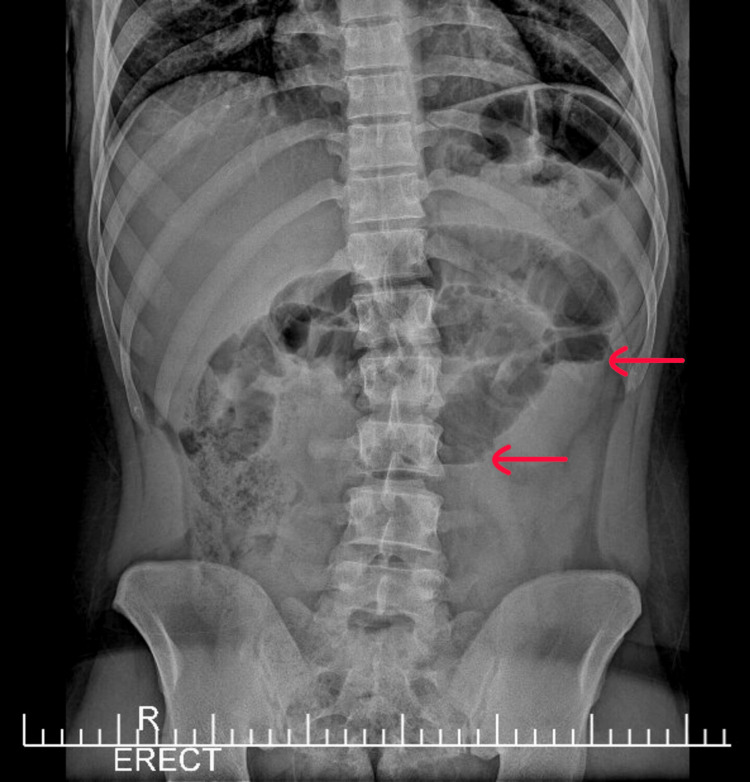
An erect X-ray abdomen showing multiple dilated bowel loops with air-fluid levels (red arrows)
